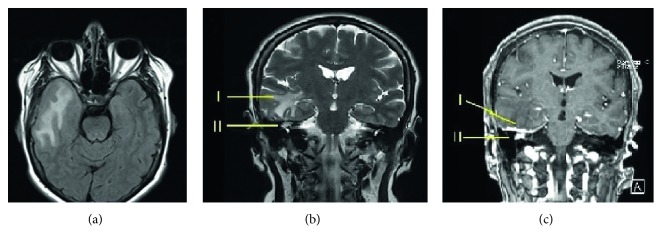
Magnetic resonance imaging of the temporal bone. (a) Axial T2 (T2-TIRM) fluid-attenuated view to suppress cerebrospinal fluid shows extensive edema of the right temporal lobe. (b) Coronal T2 image shows intracranial inflammation (I) and soft tissue located supralabyrinthine area of the right inner ear (II). (c) Coronal T1 view with gadolinium contrast shows dural thickening with contrast enhancement throughout the right tegmen (I). Soft tissue with contrast enhancement is also seen at the supralabyrinthine area (II).
